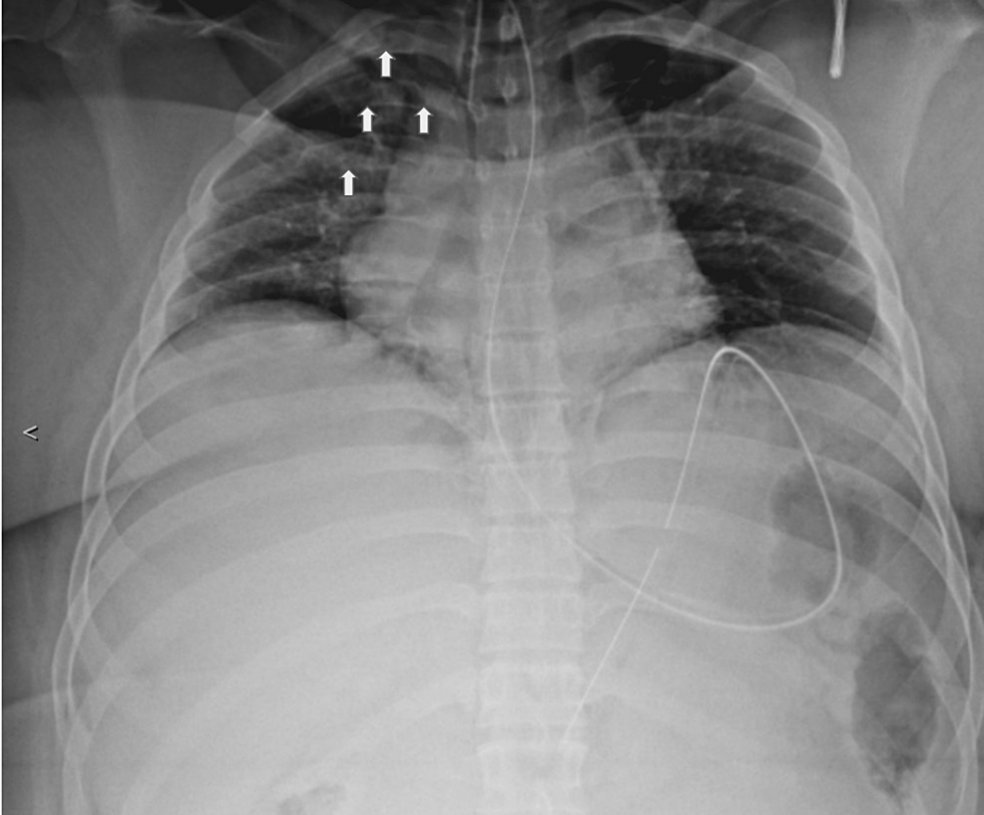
Portable chest X-ray shows fractures of the right second through fourth ribs (white arrows). Corresponding CT (Figure 1) confirmed fractures of the right first through seventh ribs, left first rib, and right L5 transverse process.
